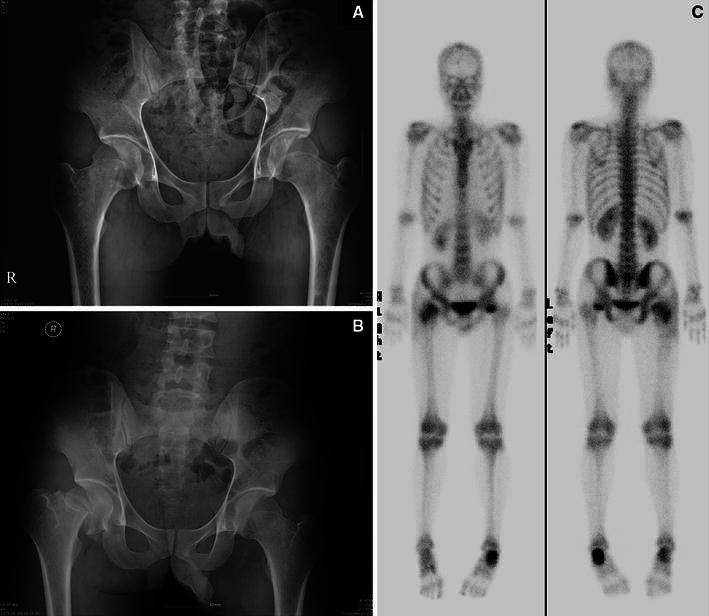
a A simple radiological examination was performed; no abnormal finding was found. b At 4 weeks after enrollment, a simple radiological examination was performed on the hip areas reported to be painful, and the radiographs obtained revealed a displaced femoral neck fracture. c A bone scan showed hot uptakes at both hips and at the left ankle joint
